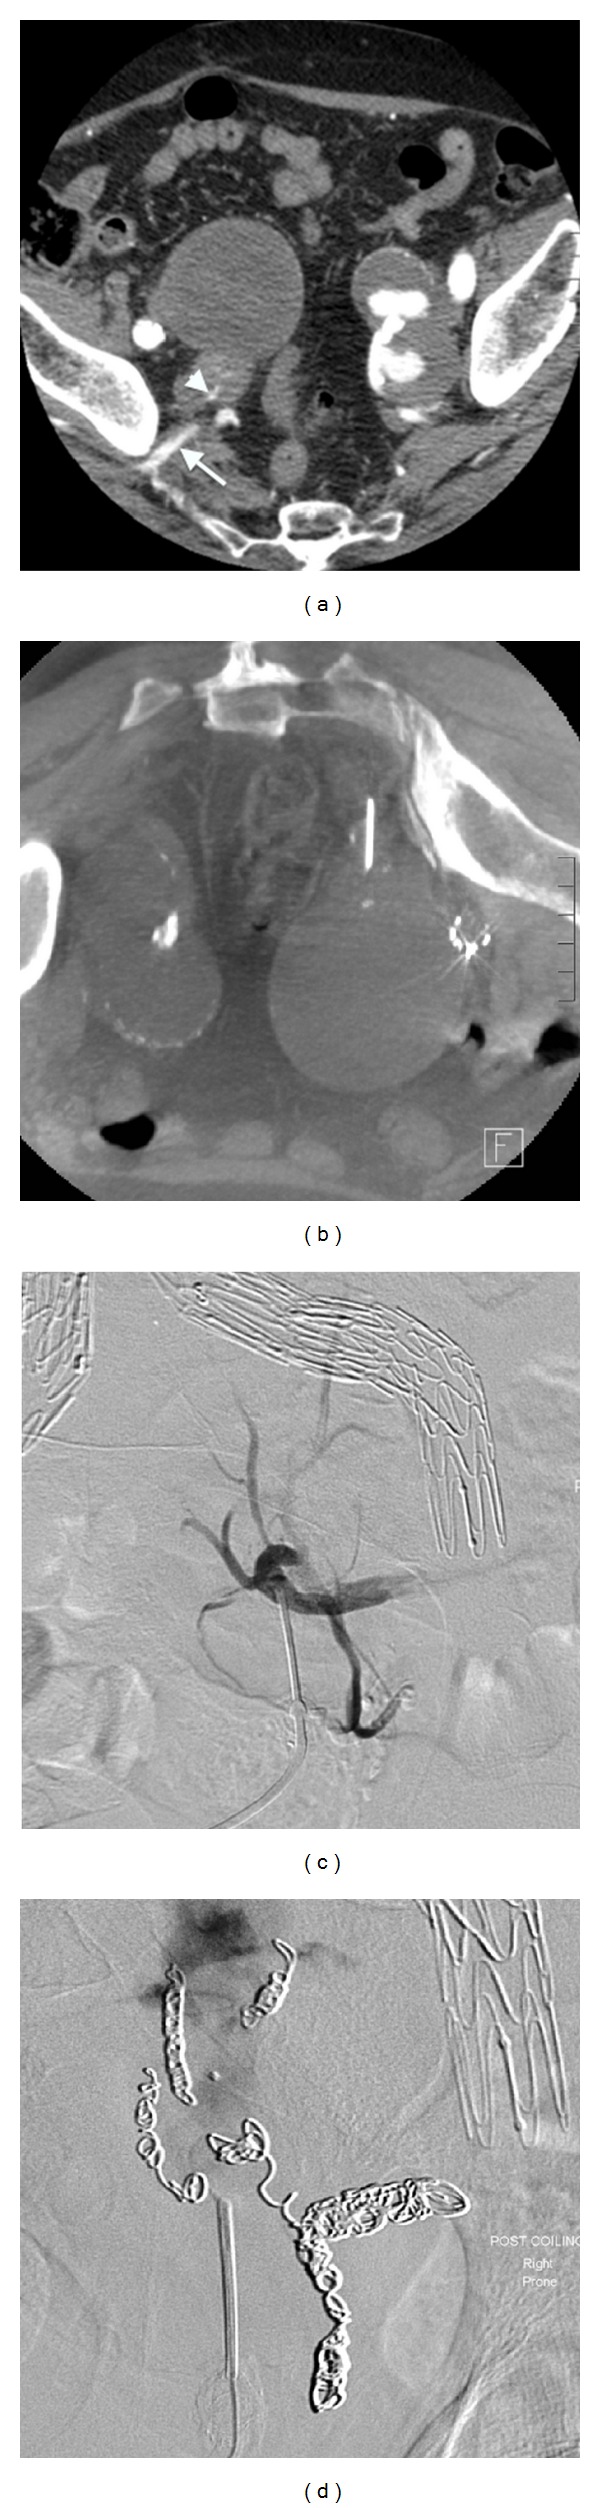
(a) Axial CT demonstrating bilateral internal iliac artery aneurysms, and contrast can be seen entering the right IIA aneurysm (*arrowhead*) via the superior gluteal artery (*arrow*). (b) Prone axial CT showing the needle cannulating the superior gluteal artery after traversing sciatic notch. (c) Prone angiogram with the needle in the superior gluteal artery demonstrating communication with five feeding vessels. (d) Angiogram postcoiling of vessels demonstrating no perfusion of aneurysmal sac of filling vessels.
